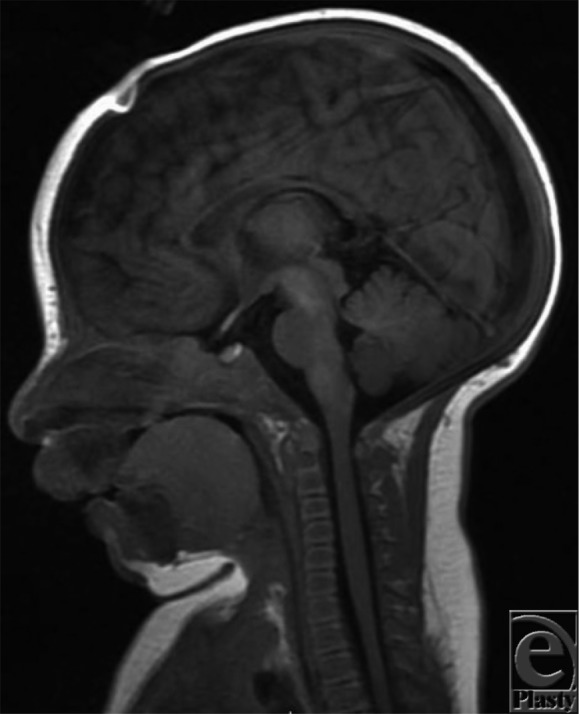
Magnetic resonance imaging, sagittal T1 FLAIR image at 2 months of age, indicating posterior displacement of the tongue causing significant pharyngeal airway obstruction.
